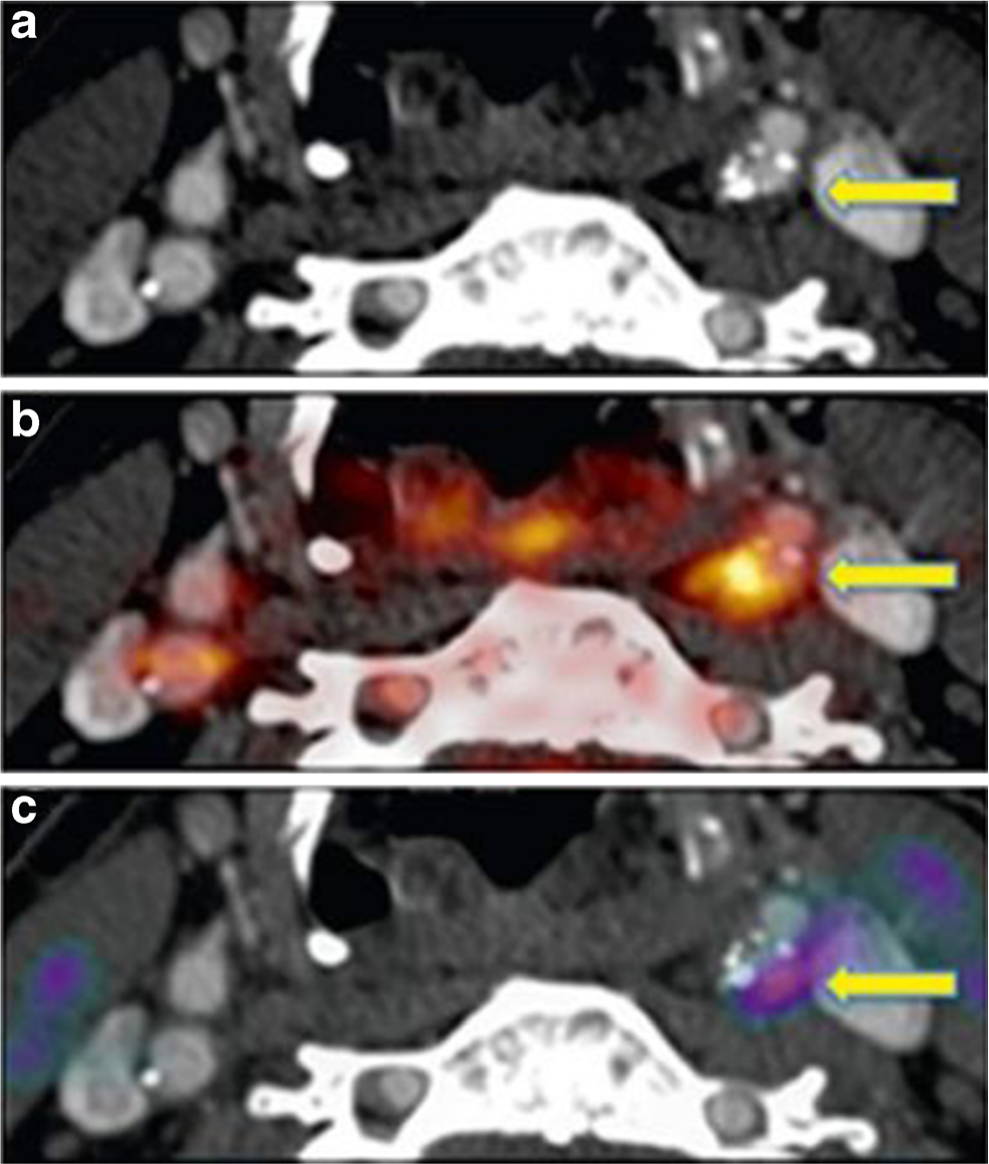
Positron emission tomography/computed tomography (PET/CT) imaging of a culprit carotid stenosis after stroke. a CT angiography. Stenosis in the left internal carotid artery (arrow). b Fused ^18^F-fluorodeoxyglucose (FDG) PET/CT. Intense uptake in the culprit lesion (arrow). c Fused ^18^F-fluoromisonidazole (FMISO) PET/CT. Corresponding uptake is indicative of intraplaque hypoxia (arrow). Reproduced from Joshi et al. [47]
